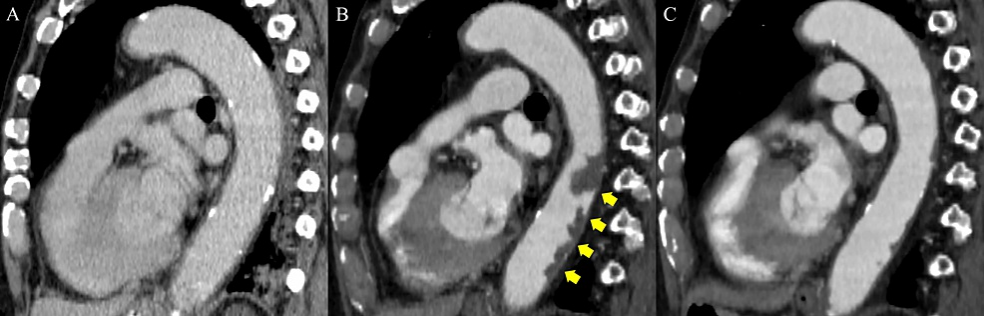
The descending aorta in contrast-enhanced CT A: CT scan upon admission, showing the descending aorta. B: CT after diagnosis of persisting HIT, revealing a mural thrombus in the descending aorta (yellow arrows). C: CT following high-dose IVIG therapy, showing near-complete resolution of the aortic mural thrombus. HIT, heparin-induced thrombocytopenia, IVIG, Intravenous immunoglobulin
